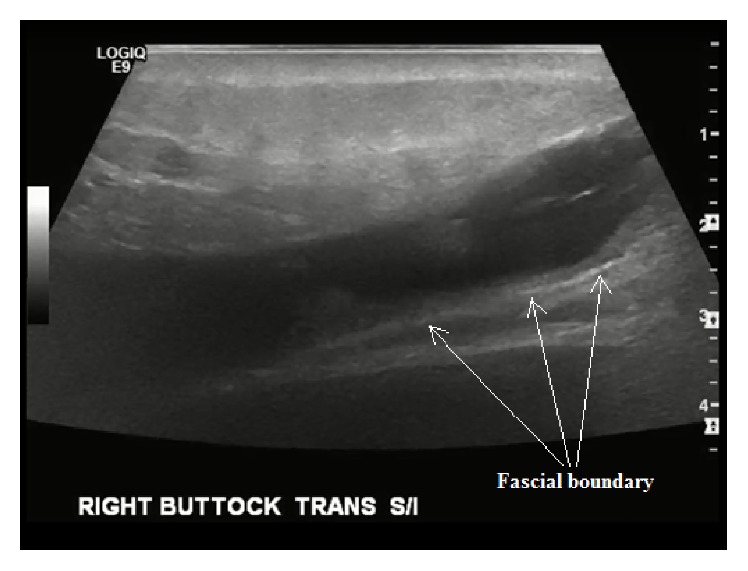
Transverse ultrasound view of lesion. Note fluid collection bounded by fascial planes.
